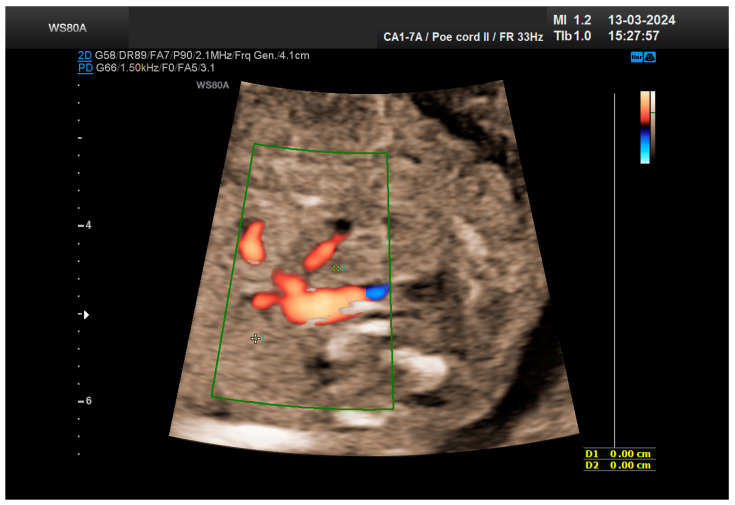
The two kidneys fused with aortic arterial vascularization. Green box—color Doppler window.
